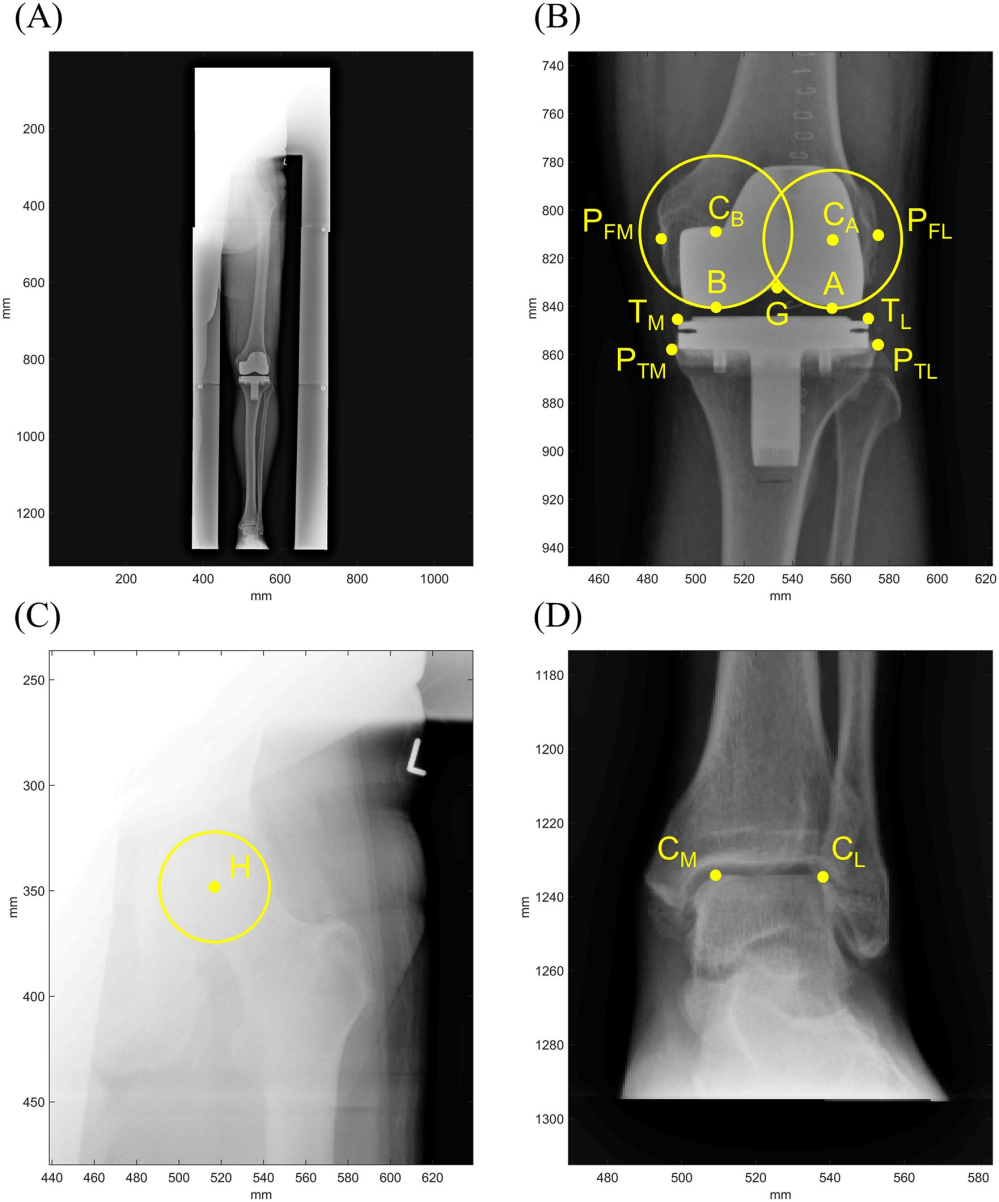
Parameter acquisition for patient-specific model adaptation (exemplary). (A) Long-leg radiograph, (B) knee region, (C) hip region, and (D) ankle region. The nomenclature is presented in Table 3.
